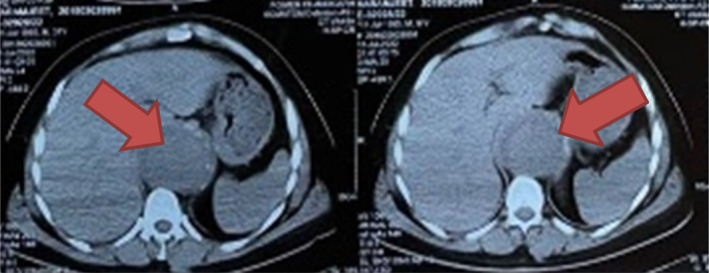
CT Aortogram Axial View showing Stanford Type B Aortic Dissection.
